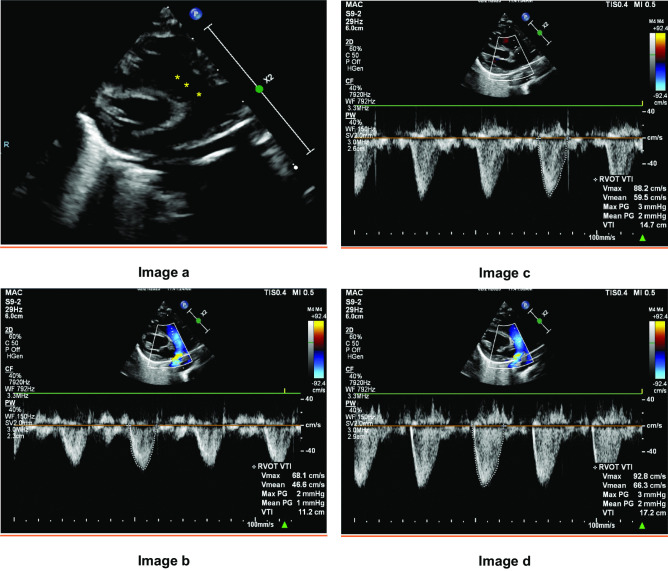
Measurement of VTI at different locations along the RVOT. a shows VTI measured at the 3 locations along RVOT in PLAX RVOT view (yellow asterisks). b–d show changes in VTI measurement as location of Doppler sampling gate changes. b shows VTI sampled below the valve. c shows VTI sampled at the hinges of valve. d shows VTI sampled at the tip of the valve. VTI, velocity time integral. RVOT, right ventricular outflow tract. PLAX, parasternal long axis view
